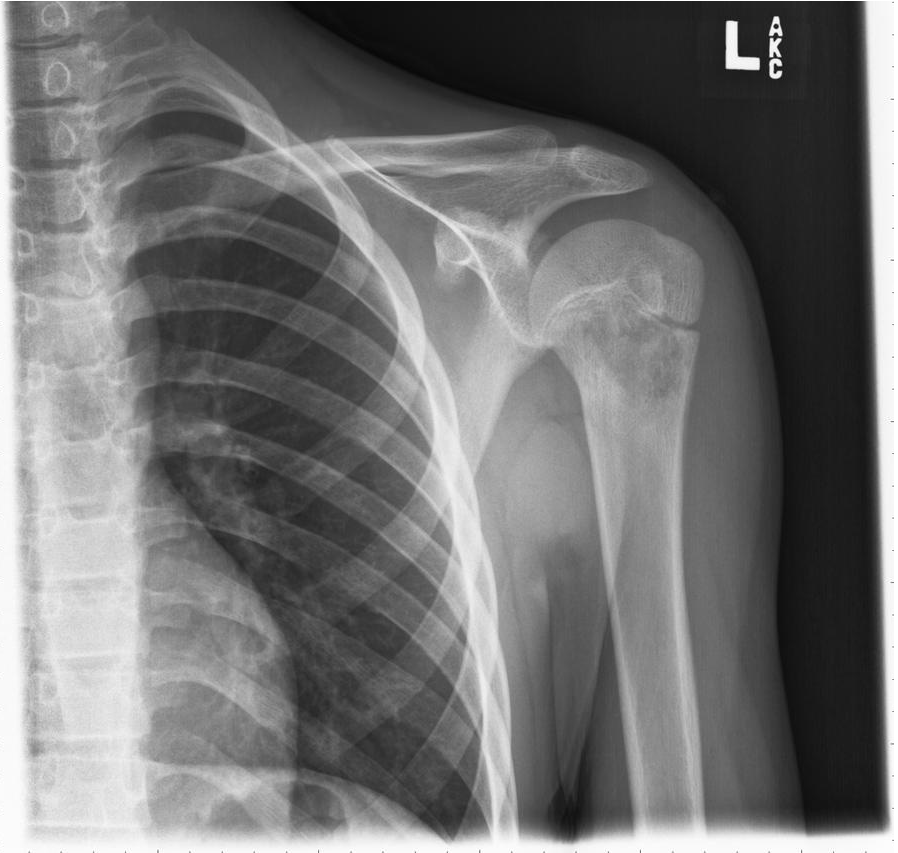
X-ray of left shoulder shows destructive lesion of humerus.
